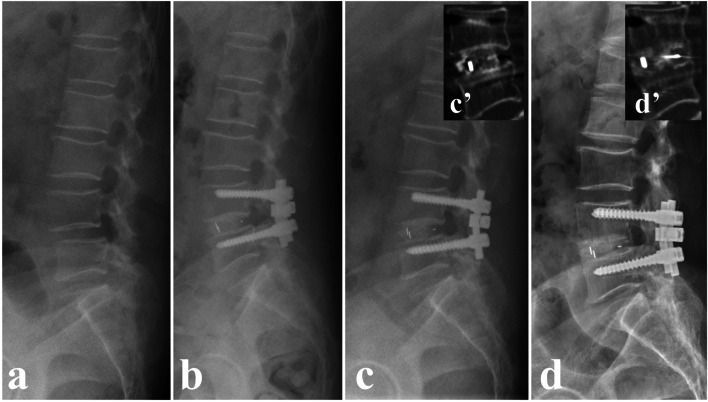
A 53-year-old woman underwent TLIF with a PEEK-Ti-HA cage. a Preoperative X-ray. b Postoperative X-ray. c X-ray at 3-month post-surgery. c’ CT scanning at 3-month post-surgery. d X-ray at the final follow-up. d’ CT scanning at the final follow-up
